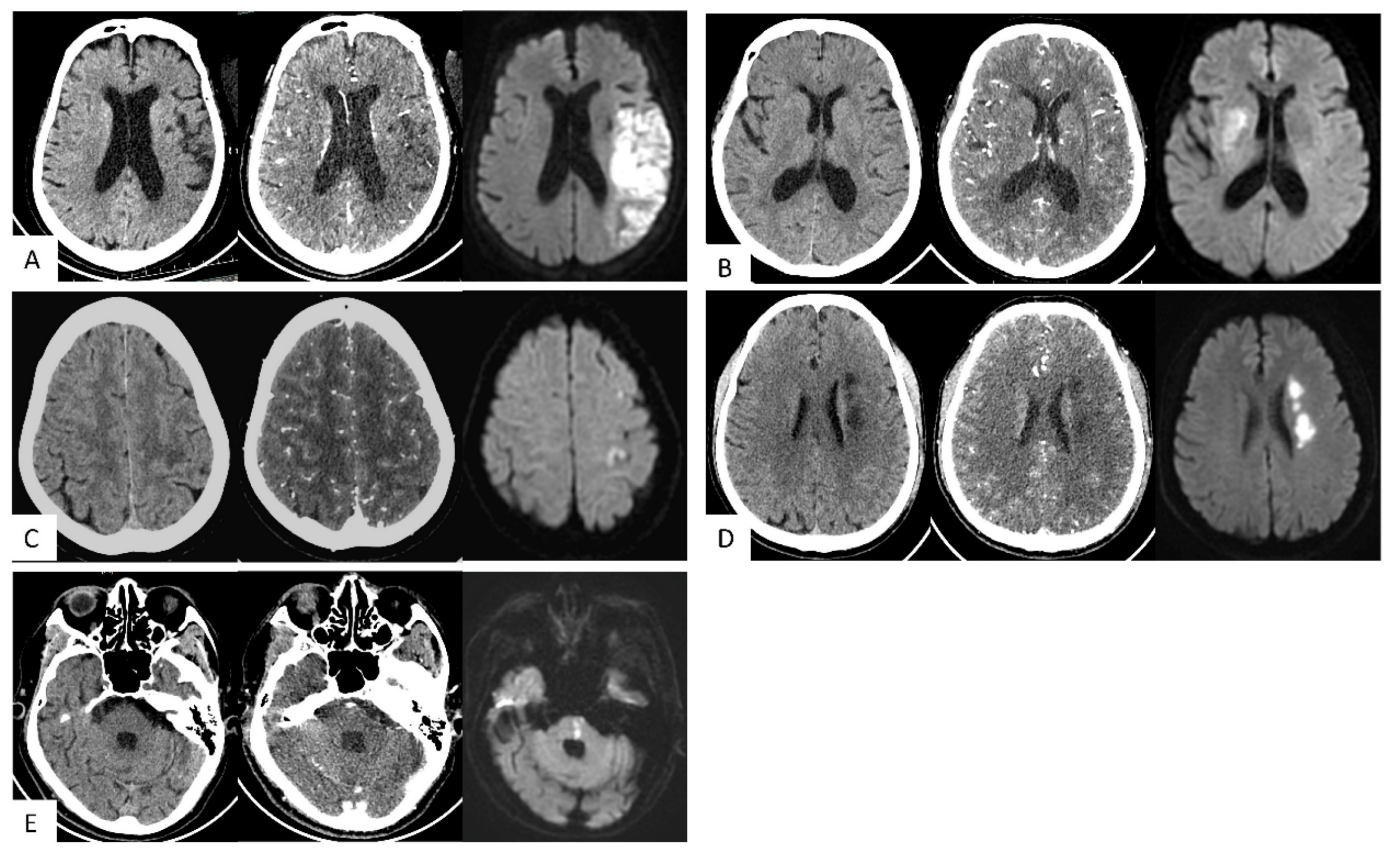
Typical cases. (A) Large vessel trunk infarction (LTI). An 85-year-old female; onset time 3+ hours. NCCT showed no obvious abnormalities. CT angiography source images (CTA-SI) revealed a large, slightly low attenuation in the left frontal and parietal lobes. The combination of CTA-SI and NCCT depicted left frontal and parietal lobe ischemic stroke. Diffusion-weighted imaging (DWI) showed a large, diffuse, restricted area in the left frontal and parietal lobes. (B) Deep perforator vessel infarction (DPI). A 67-year-old female; onset time 23 hours. NCCT showed no obvious abnormalities. CTA-SI revealed a slightly low attenuation in the right basal ganglia. The combination of CTA-SI and NCCT depicted right basal ganglia ischemic stroke. DWI showed a patchy diffuse restricted area in the right basal ganglia. (C) Superficial perforator vessel infarction (SPI). A 67-year-old female; onset time 24 hours. NCCT showed a slightly low attenuation in the subcortical region of the left parietal lobe. CTA-SI showed no obvious abnormalities. The combination of CTA-SI and NCCT did not indicate the infarct focus. DWI showed a patchy and punctate restricted area in the subcortical region of the left frontal and parietal lobe. (D) Watershed infarction (WI). A 61-year-old male; onset time 3+ hours. NCCT and CTA-SI demonstrated chainlike low attenuation in the left paraventricular region, and there were no signs of old lesions in the surrounding area. DWI showed a chainlike restricted area in the left paraventricular region. (E) Infratentorial infarction (IF). A 78-year-old male; onset time 1+ hours. NCCT and CTA-SI showed no obvious abnormalities in the posterior fossa. Beam hardening artifacts were shown on CTA-SI. DWI showed patchy restricted area in the pons which located in the posterior fossa.
